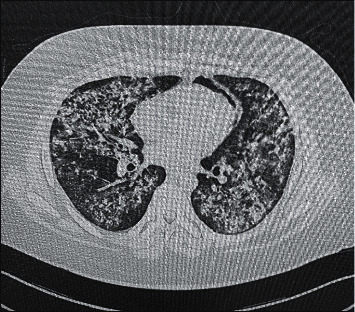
Pretreatment high resolution computed tomography.
